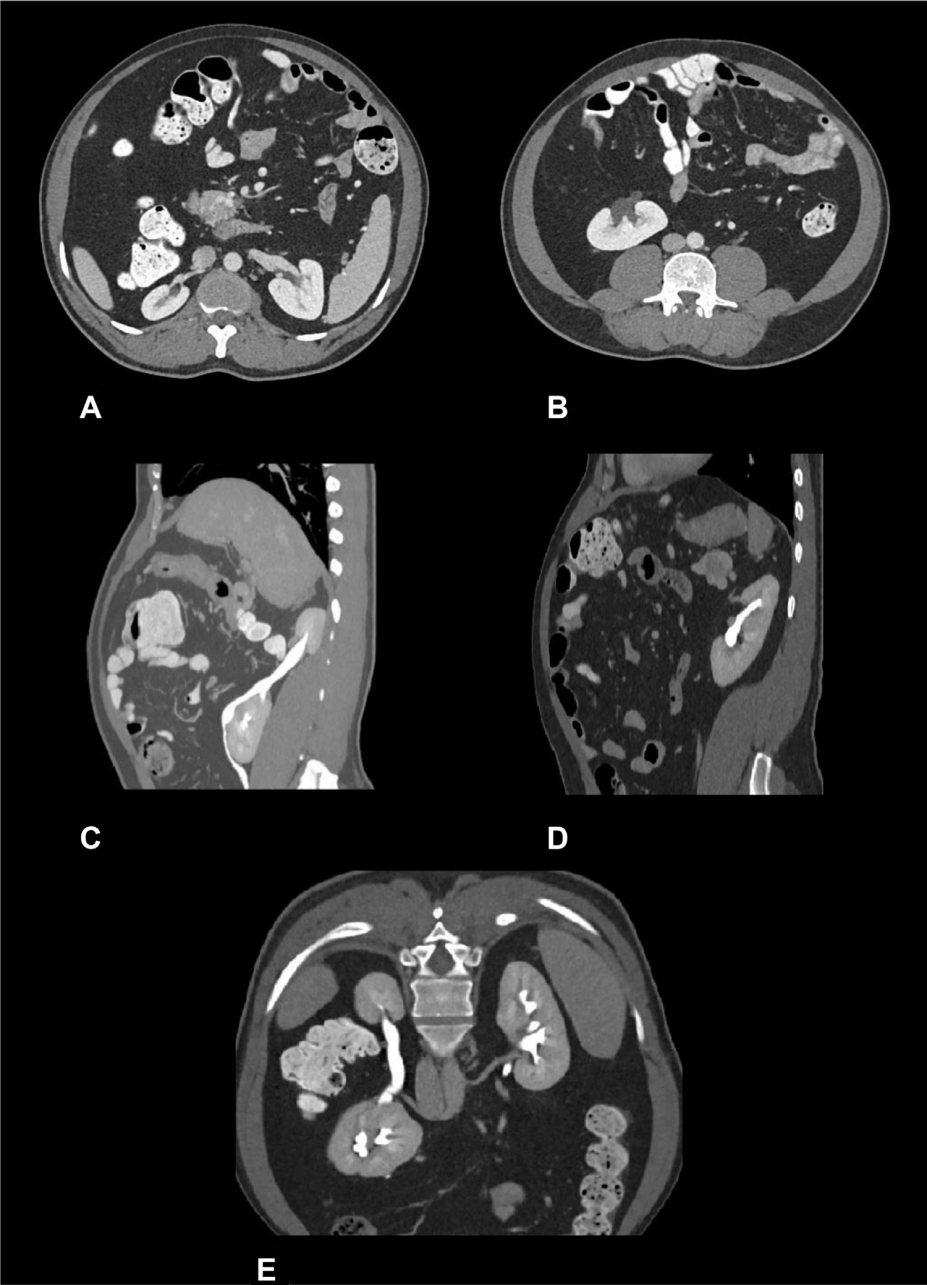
Selected reconstruction images of CT abdomen and pelvis study with intravenous venous acquired contrast. (A and b) show on axial view the 3 kidneys on the abdominal region. (C) Demonstrates on sagittal view the 2 kidneys on the right hemiabdomen, the cranial kidney is smaller, and (D) shows a native left kidney. (E) Coronal view of CT abdomen shows the 3 kidneys.
